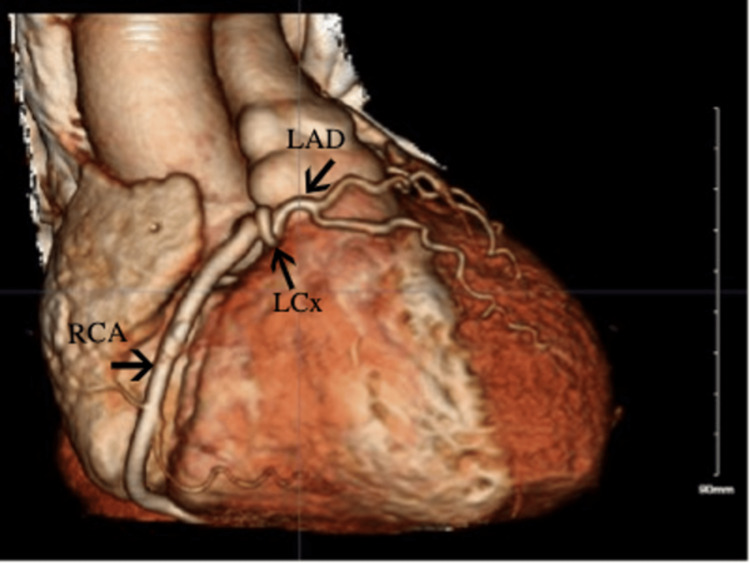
Three-dimensional (3D) image in the coronary computed tomography angiography (CCTA) demonstrating an anomalous coronary artery
